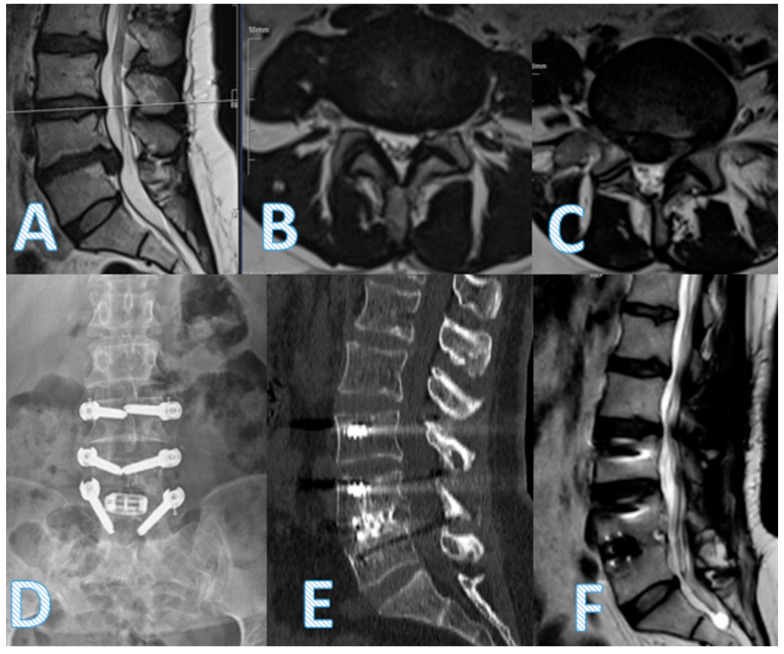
Preoperative sagittal (A) and axial MR images at the L3–4 (B) and L4–5 (C) levels demonstrate severe foraminal stenosis and compression of the exiting nerve roots at L4–5, more prominent on the right, consistent with the patient’s symptoms of neurogenic claudication and bilateral leg pain. Postoperative anteroposterior X-ray (D), sagittal CT (E), and sagittal MR (F) images show decompression and stabilization achieved through L4–5 transforaminal lumbar interbody fusion (TLIF) using a PEEK rod and interbody cage, with restored disk height and adequate neural decompression.
